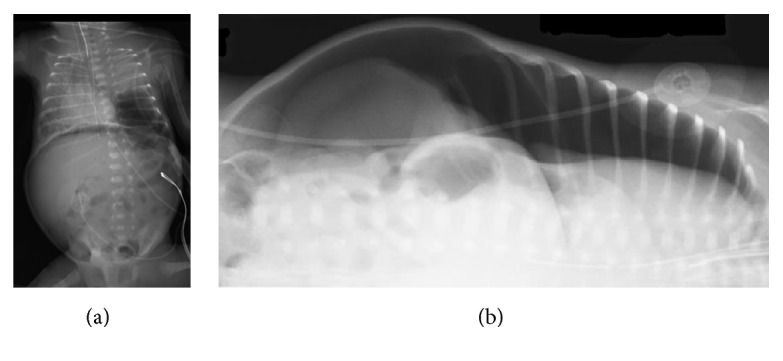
(a) Radiograph of chest and abdomen shows pneumothorax, pneumoperitoneum, and cystic lesion in the left lower lobe. (b) Right lateral decubitus view of chest and abdomen shows left sided pneumothorax with pneumoperitoneum.
